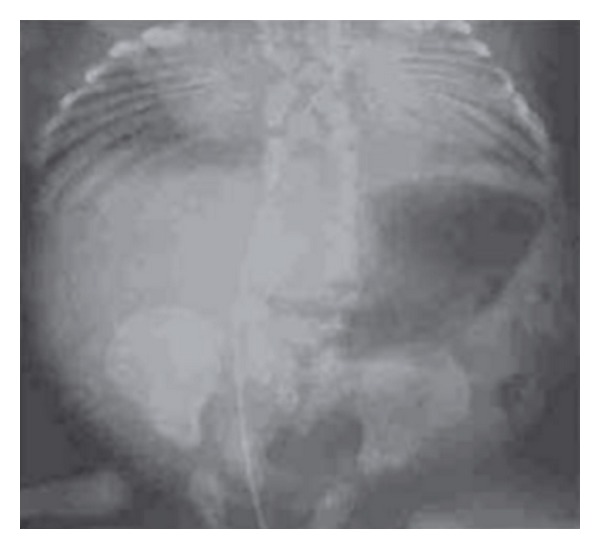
Radiograph features of spondylothoracic dysostosis demonstrating contiguous vertebral malformations with symmetric posterior rib fusion. Reproduced with permission Expert Opinion in [9].
